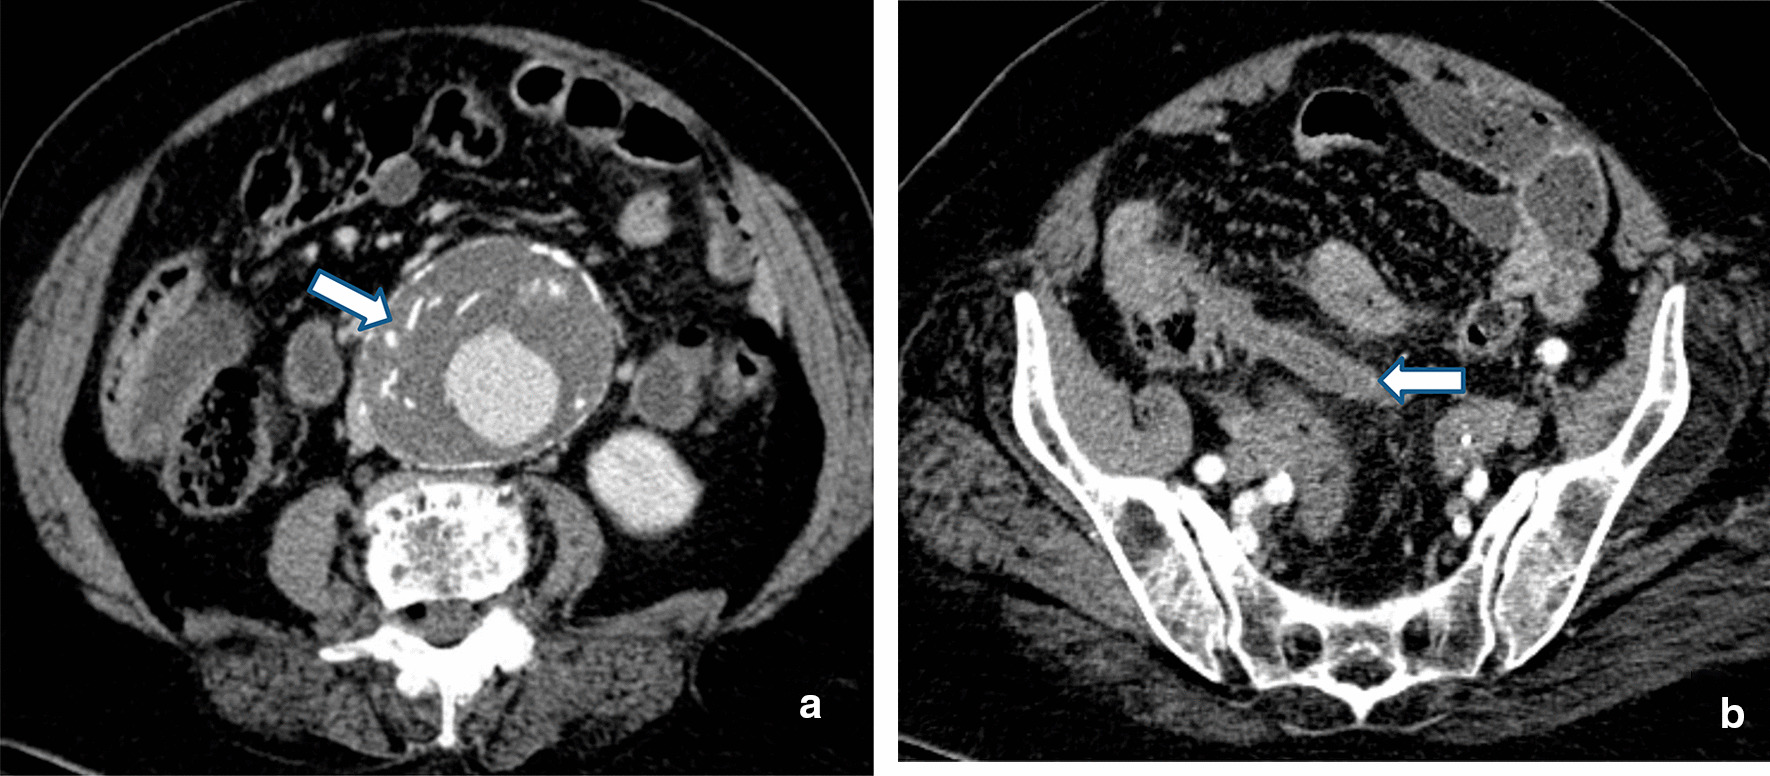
Preoperative computed tomography (transverse sections): a Aortic abdominal aneurysm of 8 cm (antero-posterior) × 8.5 cm (transversal), without signs of rupture (no free liquid or signs of retroperitoneal hematoma are visible), and radiological signs of intrathrombal hemorrhage (arrow). b Thickening of the sigmoid wall and slight increase in the echogenicity of the adjacent fat (5 cm in length). Inflamed appendix and increased echogenicity of adjacent fat (arrows)
